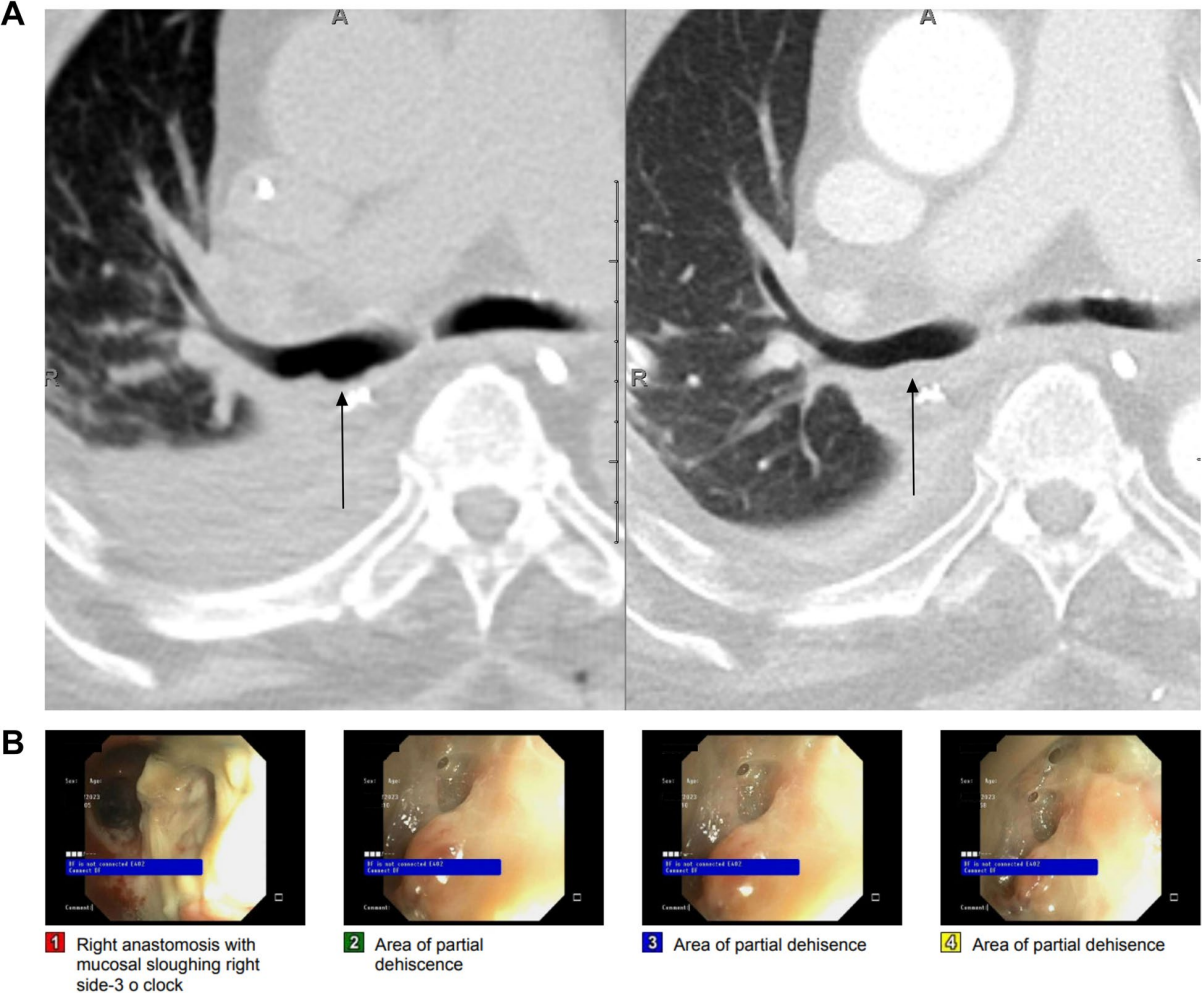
Partial bronchial anastomotic dehiscence in a 71-year-old male. A Comparison axial CT images (lung windows) show an abnormal contour of the posterior right bronchial anastomosis and membranous portion of the perianastomotic region (left, black arrow) 2 weeks after bilateral lung transplantation, which was new from the previous scan obtained 1 week after transplant with a normal appearing anastomosis (right, black arrow). There was no extraluminal air or pneumomediastinum. B Bronchoscopy performed just prior to the left image in (A) revealed membranous dehiscence which was 0–25% circumferential and ischemia/necrosis which was 51–100% circumferential, prompting the CT
